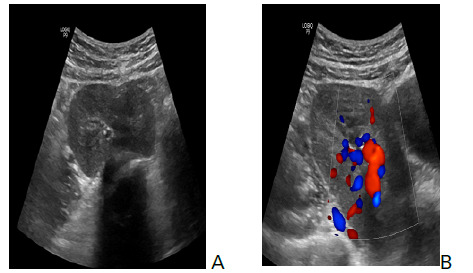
Ultrasound images showing both kidneys fused together within the pelvic cavity (A). Color Doppler examination revealed single renal vessels within the single renal pelvis suggestive of pancake appearance (B).
